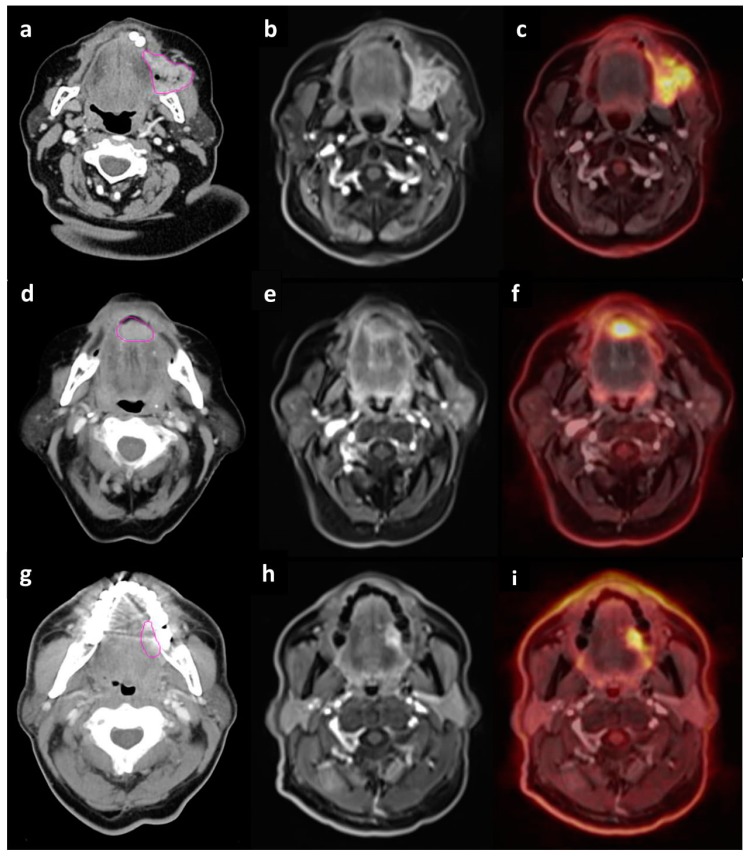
Contrast enhancement computed tomography (CT) (a,d,g), T1-weighted volumetric interpolated breath hold examination (VIBE) magnetic resonance (MR) (b,e,h) and positron emission tomography/MR (PET/MR) (c,f,i) imaging of head and neck cancer patients. (a,b,c)—patient with squamous cell carcinoma of the buccal mucosa in clinical stage T3N2b; (d,e,f)—patient with squamous cell carcinoma of the floor of the mouth in clinical stage T1N0; (g,h,i)—patient with squamous cell carcinoma of the oral tongue in clinical stage T2N0.
